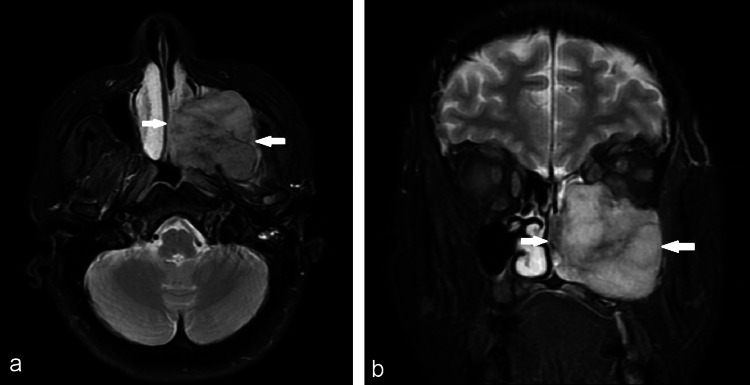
Magnetic resonance imaging of the same patient (T2 sequence). (a) Axial view; (b) Coronal view (a) axial view; (b) coronal view
